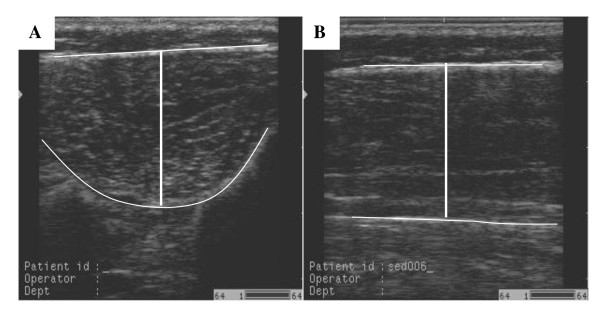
Ultrasound images of anterior tibial muscle group, showing line of thickness measurement. A: Transverse scan. B: Longitudinal scan
